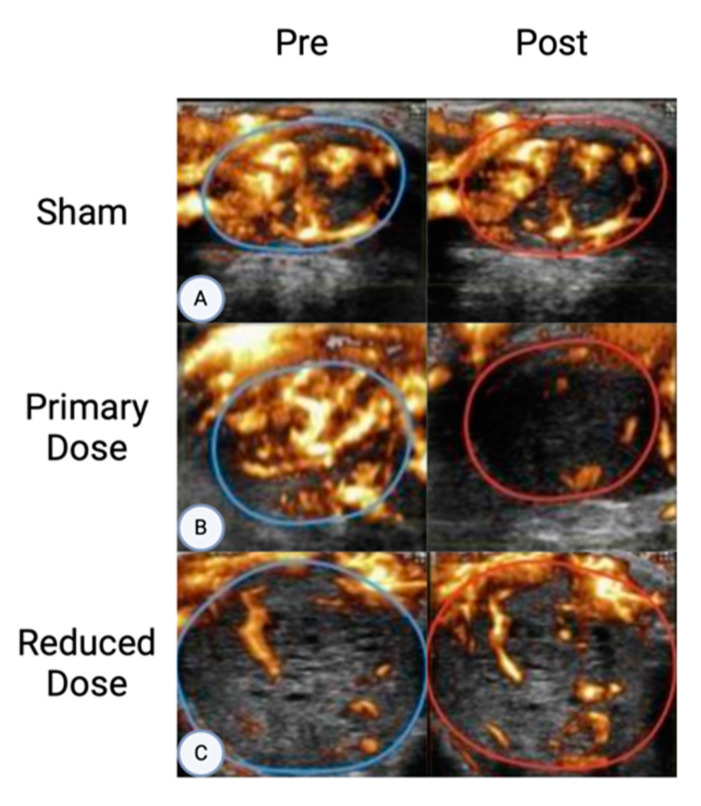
Power Doppler images pre- and post-AVUS adapted from D’Souza et al. show dose-dependent responses of AVUS within targeted areas (indicated by circle regions of interest) [5]. The sham treatments (A) show equivalent perfusion before and after therapy. After treatment of a primary dose (B), a significant reduction in tumor perfusion can be visualized. Reduced-dose therapy (C), while showing a variable response overall, showed an increased perfusion in the majority of cases.
